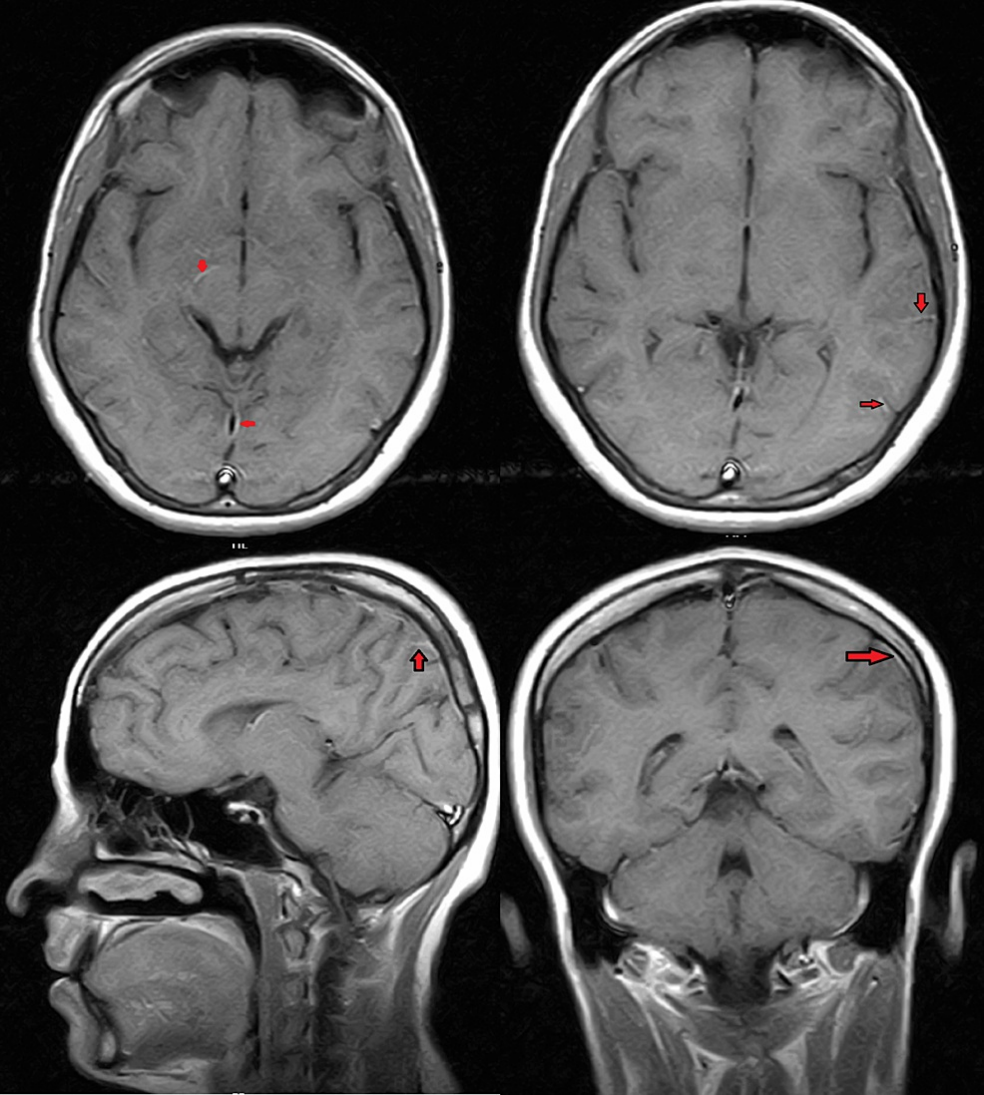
Post-contrast T1-weighted images show mild leptomeningeal enhancement (shown by red arrows).
